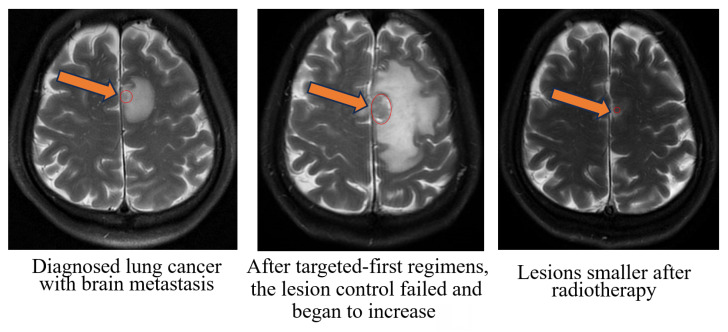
Radiographic images of a typical patient with gross tumor volume (GTV at the mark).
